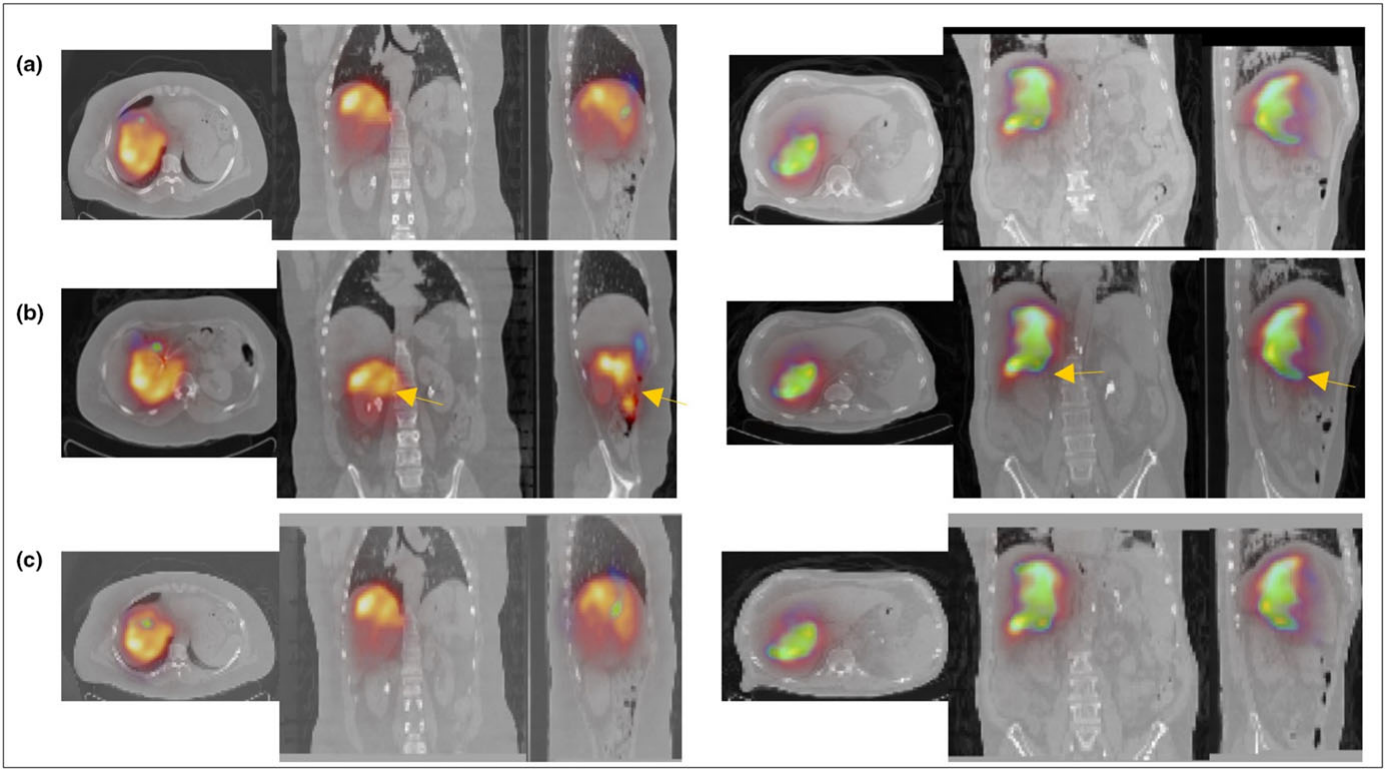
Patient 6 (left) and 8 (right) co‐registered computed tomography (CT) and single photon emission tomography (SPECT) images of 99mTc‐MAA (blue) and 90Y microsphere (red) for reference images of CT of post‐treatment (a), CT‐CT registration (b) and 90Y SPECT (c). The arrows in (b) show the misalignment between the CT and SPECT images showing activity distributions outside of the liver boundary in the coronal and sagittal views.
